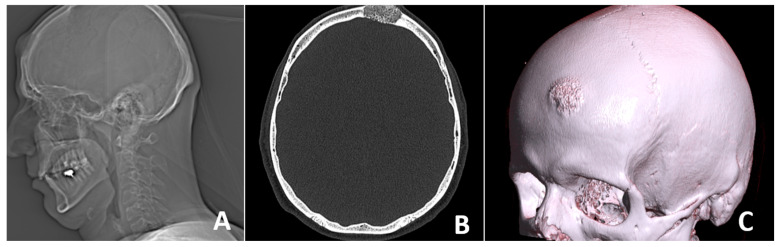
(A) X-ray and (B,C) CT scan prior to surgery reveal a bone lesion in the left frontal bone, involving the diploe, with a homogeneous structure, spiculated anterior contour, and local bone expansion.
